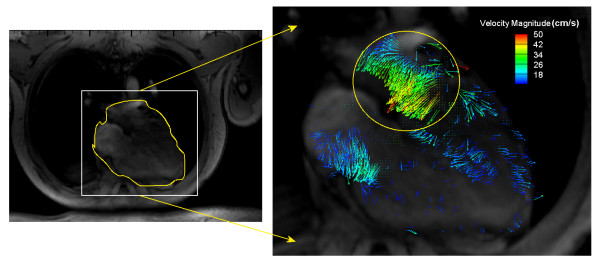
One of the heart image slices using the 4-D Phase contrast (PC)-MRI system at 256 × 192 resolution. The color indicates its velocity magnitude.
